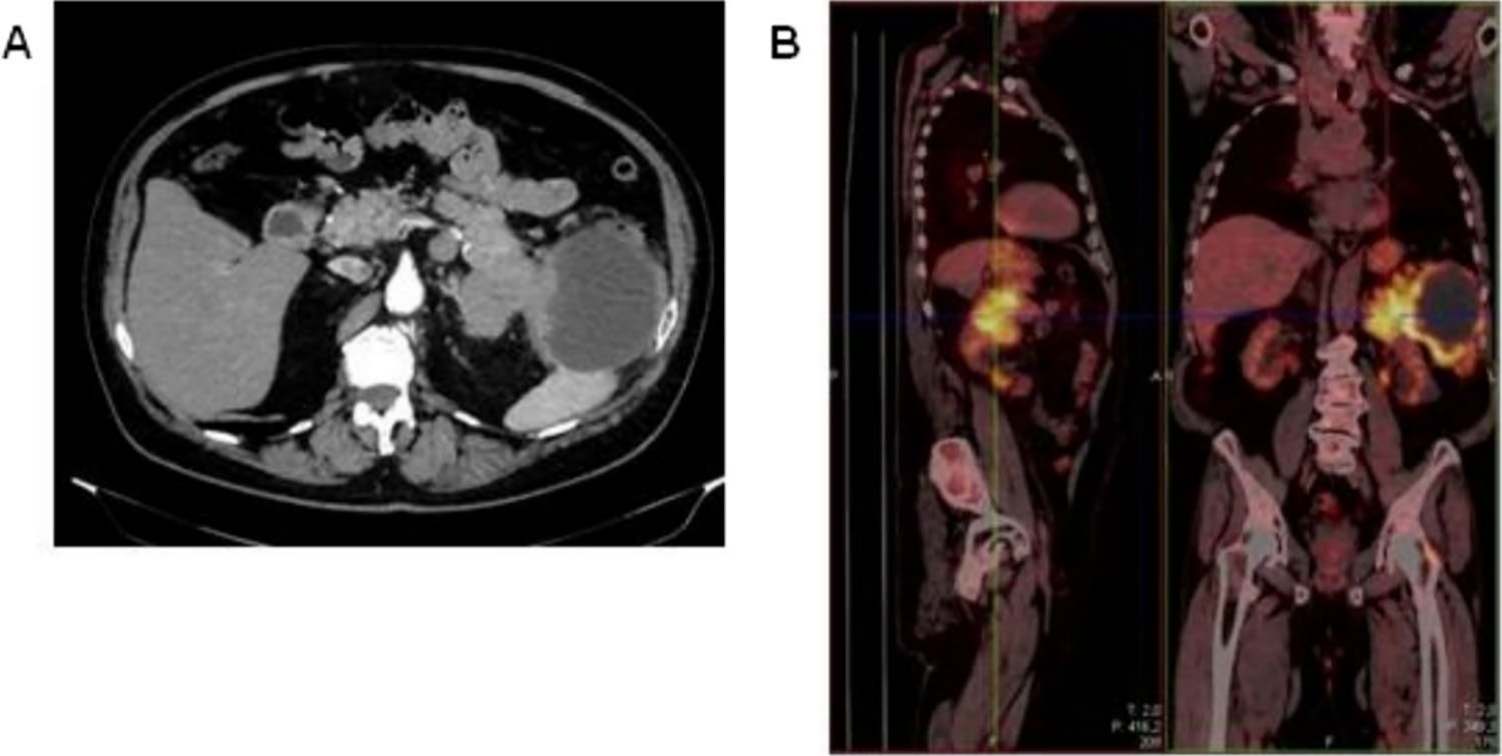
CT (A) and PET (B) scans at baseline
